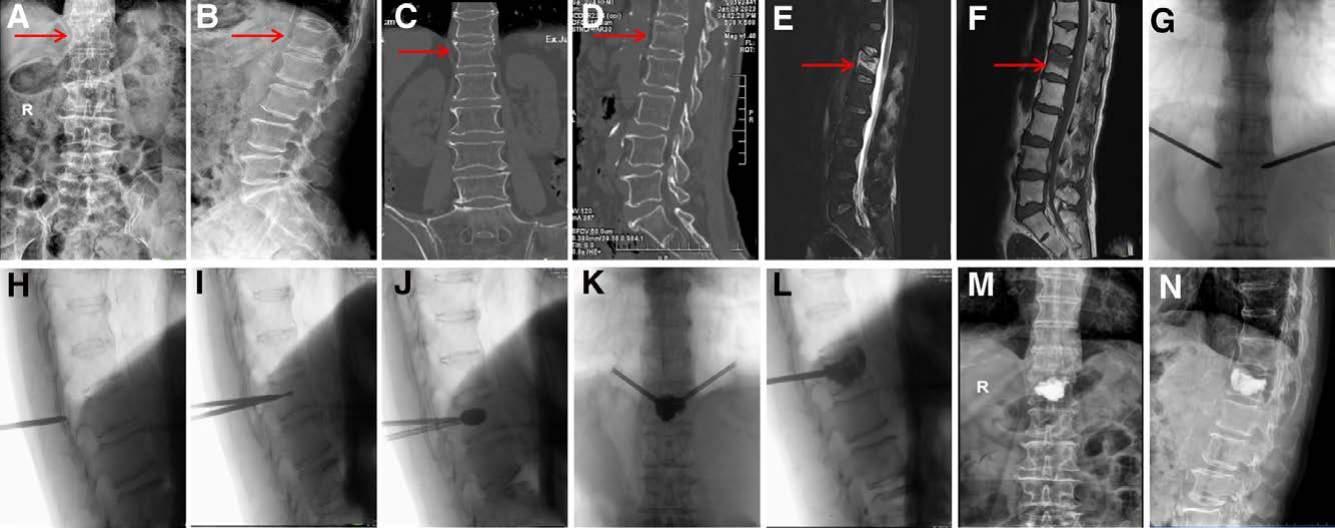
(A and B) X-ray examination suggests L1 vertebral compression; (C and D) CT scan shows a fracture line beneath the upper endplate of the L1 vertebral body; (E) MR sagittal T2-weighted image indicates high signal changes at the fracture site and adjacent bone (bleeding); (F) MR sagittal T1-weighted image indicates low signal changes at the fracture site and adjacent bone (bleeding); (G–I) first, the needle is inserted into the outer walls of both pedicles, followed by slow insertion of the channel into the vertebral body. A bone drill is then inserted to the anterior one-third of the vertebral body; (J–L) After balloon dilation to restore vertebral height, PMMA bone cement is slowly injected; (M and N) Postoperative X-ray in the anteroposterior and lateral views shows restored vertebral height with no obvious bone cement leakage. CT = computed tomography, PMMA = polymethylmethacrylate (bone cement).
